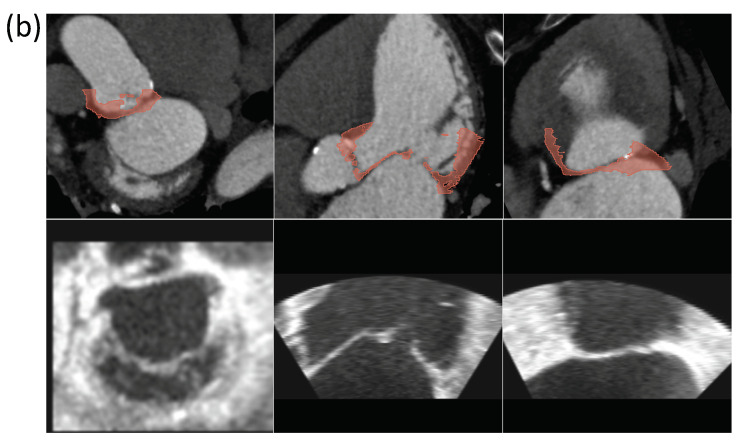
The CT/US registration results for patients CB1 (a) and CB2 (b) in axial, coronal, and sagittal view. The upper row shows the overlapping of CT and MV segmentation from the US for each plane. The bottom one shows the respective US planes.
